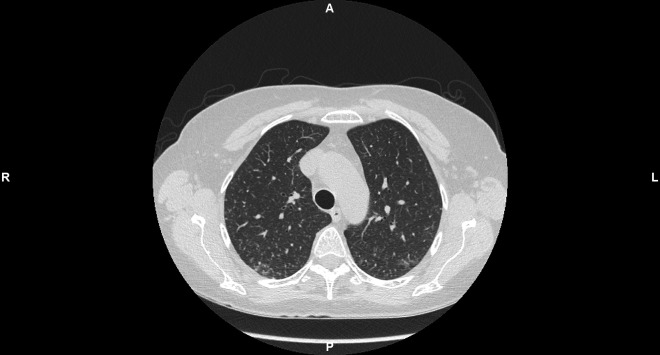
High-resolution computed tomography chest image showing numerous small pulmonary nodules in the lung apices bilaterally
